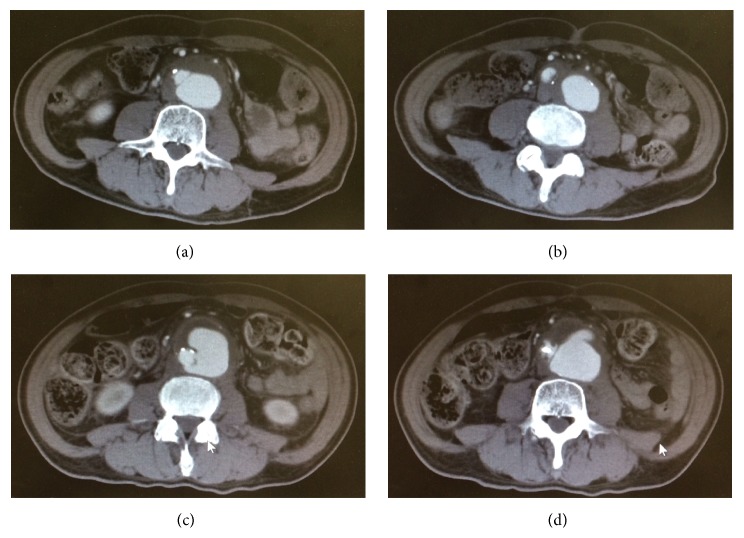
(a) and (b) Computed tomography scans taken during the patient's initial examination. (c) and (d) Computed tomography scans taken on day 9 of the illness. We observed enlargement of the abdominal aortic aneurysm and thickening of the arterial wall.
